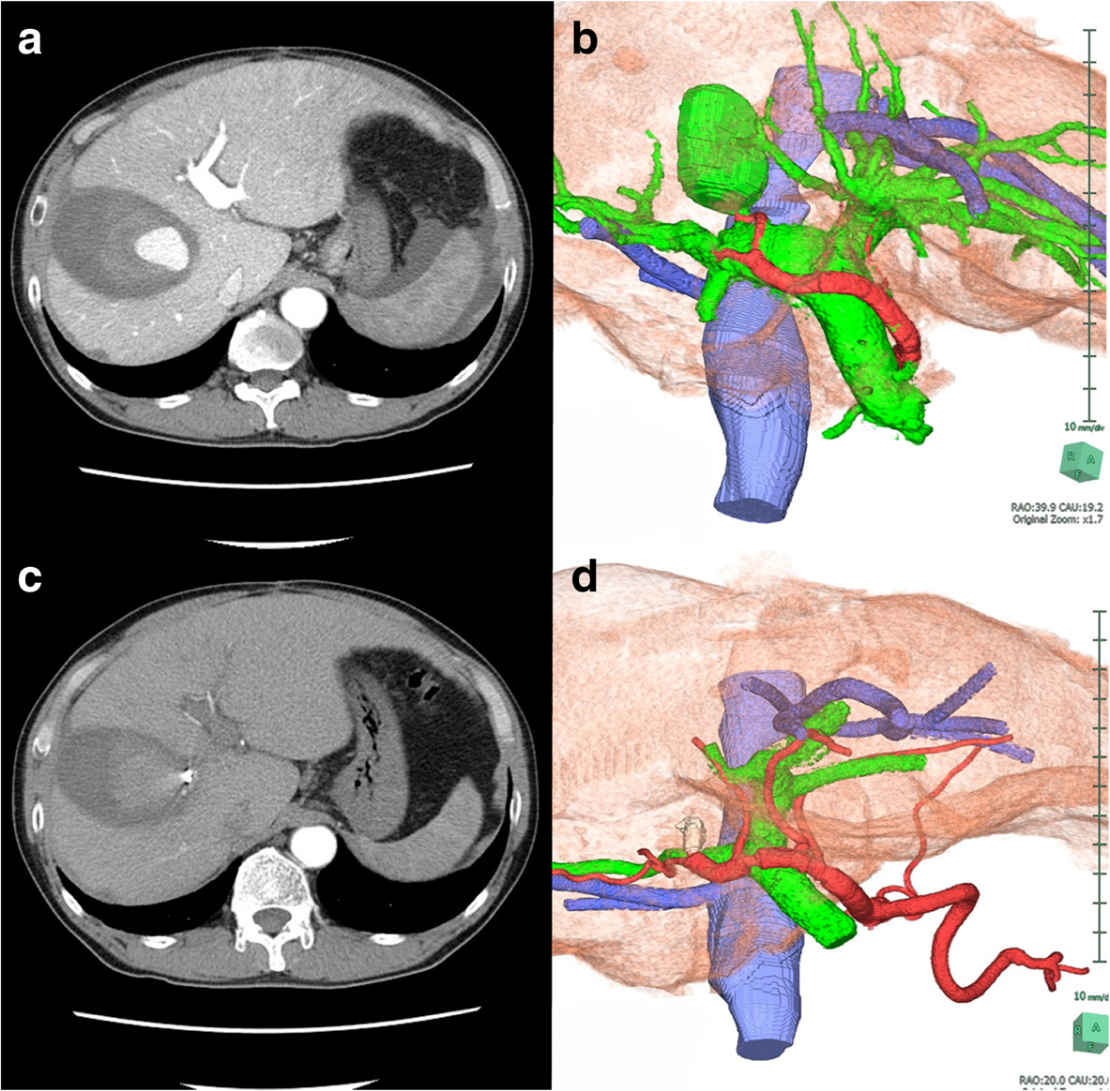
Imaging findings before and after IVR. a, b Imaging findings before IVR. Prior to IVR, the APF and PVA were detected by a dynamic CT and b 3D images. c, d Imaging findings after IVR. Three days after embolization, c dynamic CT and d 3D images clearly demonstrated both perfect interruption of the APF and disappearance of the PVA
